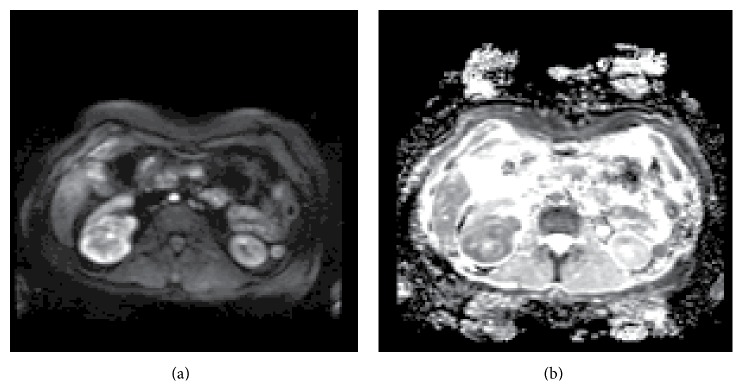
Diffusion-weighted MRI. The DWI (a) and ADC (b) images of the same patient were acquired in the axial direction using a *b* value of 800 s/mm^2^. This high-grade clear-cell RCC appears hyperintense on DWI, showing restricted diffusion (a). This was confirmed by hypointensity on the ADC map (b).
